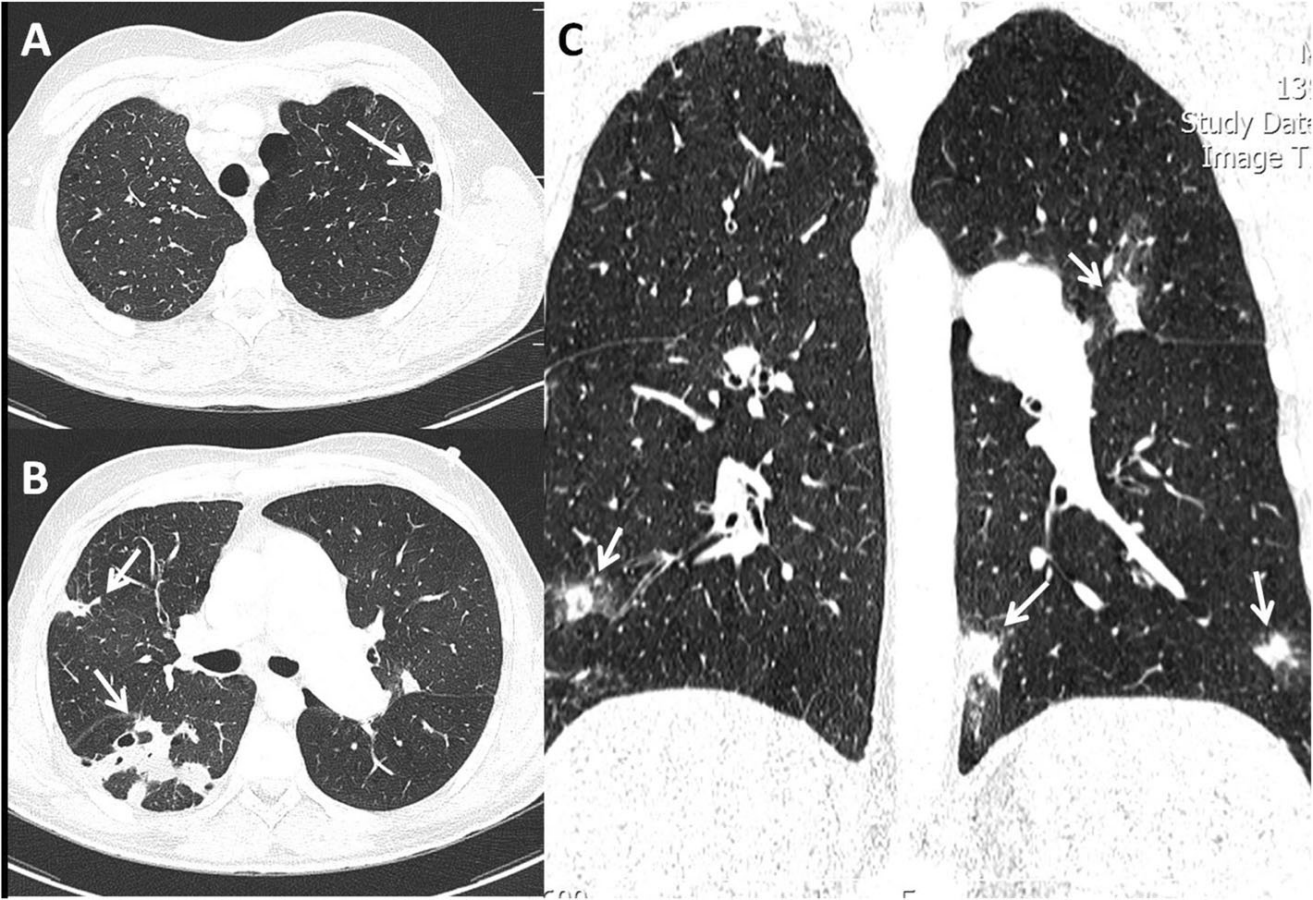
a-c axial and reconstructed coronal chest CT scan (lung window): multiple ill-defined pulmonary nodules with peripheral ground-glass halo and cavitary changes at peripheral zone of both lungs (white arrows) compatible with septic emboli
